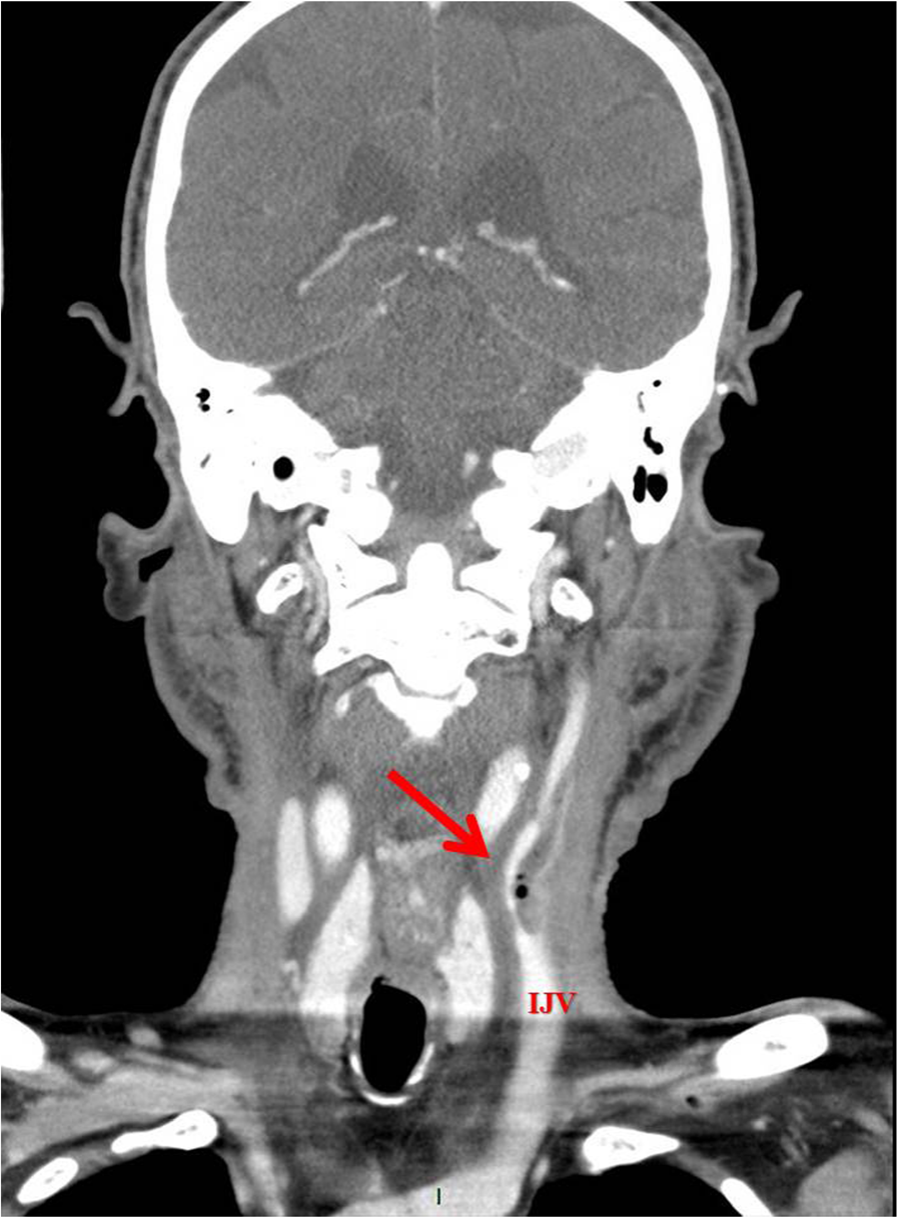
Neck computed tomography (coronal view) revealed a fistula between the left internal jugular vein and pharynx
